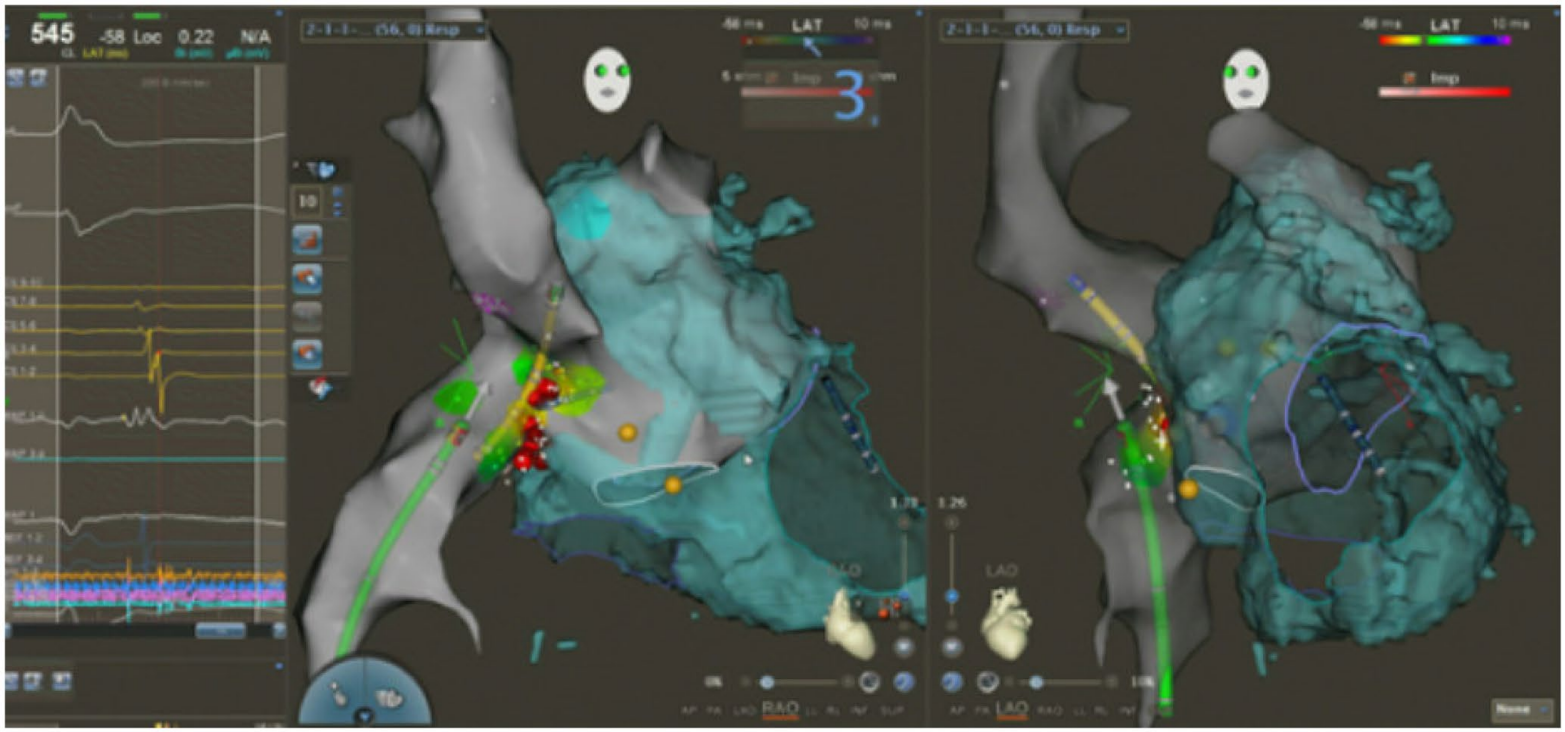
Patient with mustard operation for D‐transposition of the great arteries, successful ablation across the baffle for atrial flutter, aided by intracardiac echocardiography.
